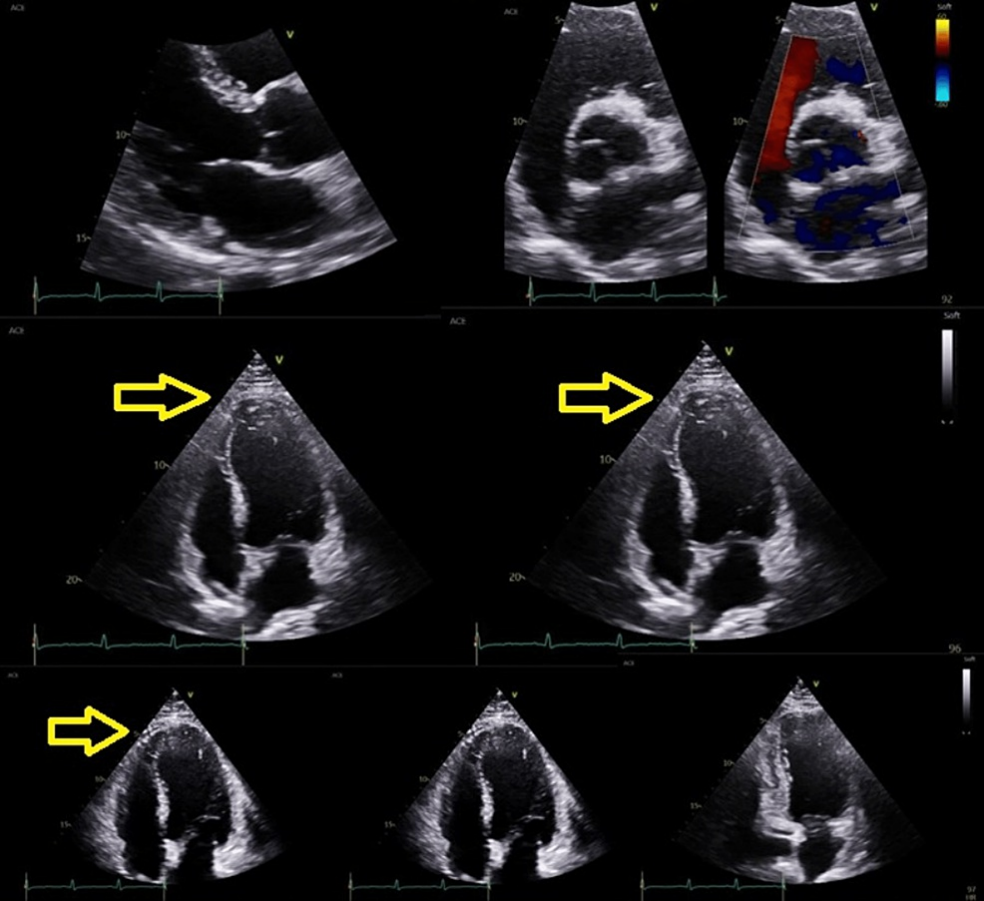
Echocardiography performed on the third day of admission shows mild nonspecific myopericardial changes (yellow arrows), normal valve thickness, and LV cavity. LV: left ventricle.
